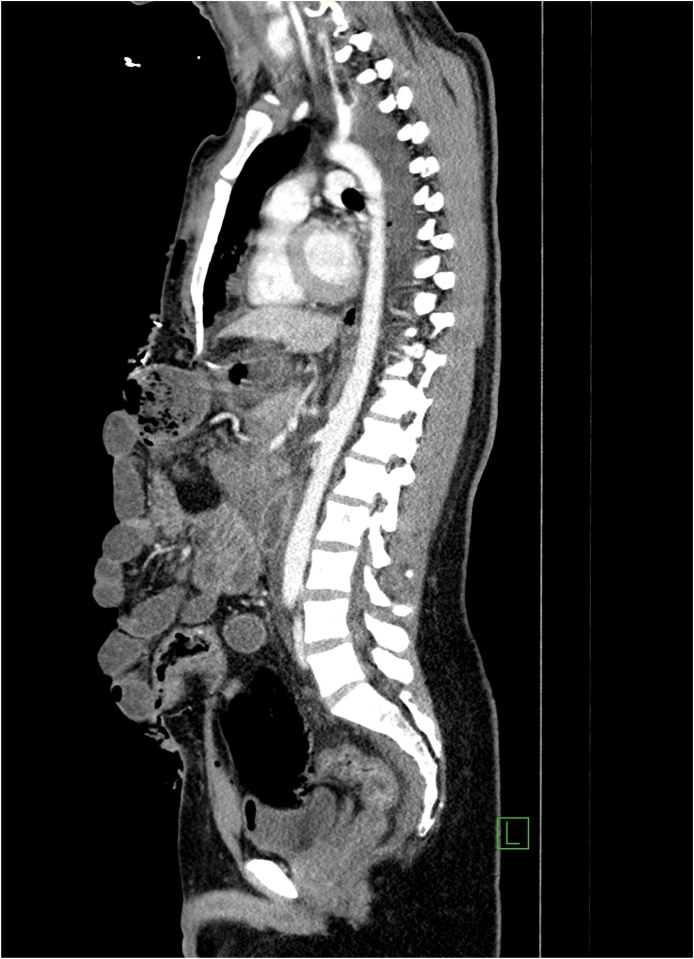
Sagittal enhanced CT of the abdomen showing anterior abdominal wall defect with multiple bowl loops evisceration.
